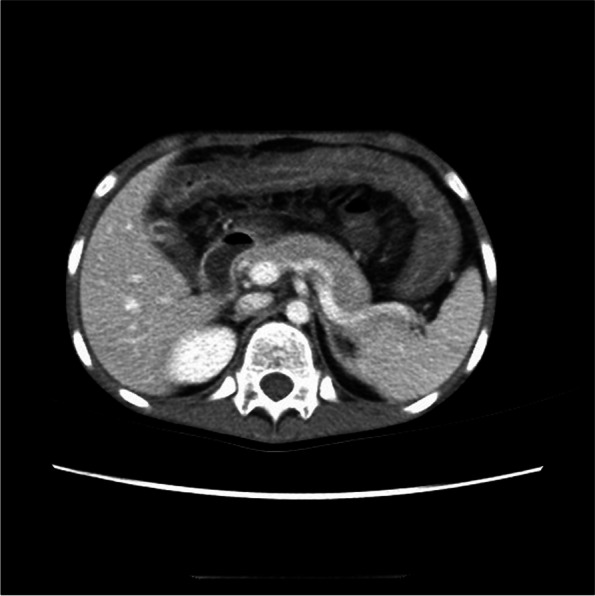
Intestinal wall thickening in LMV case. The mucosa, muscularis propria, and serosa of the intestinal wall were significantly enhanced, and the submucosa edema was significant in abdominal-enhanced CT
